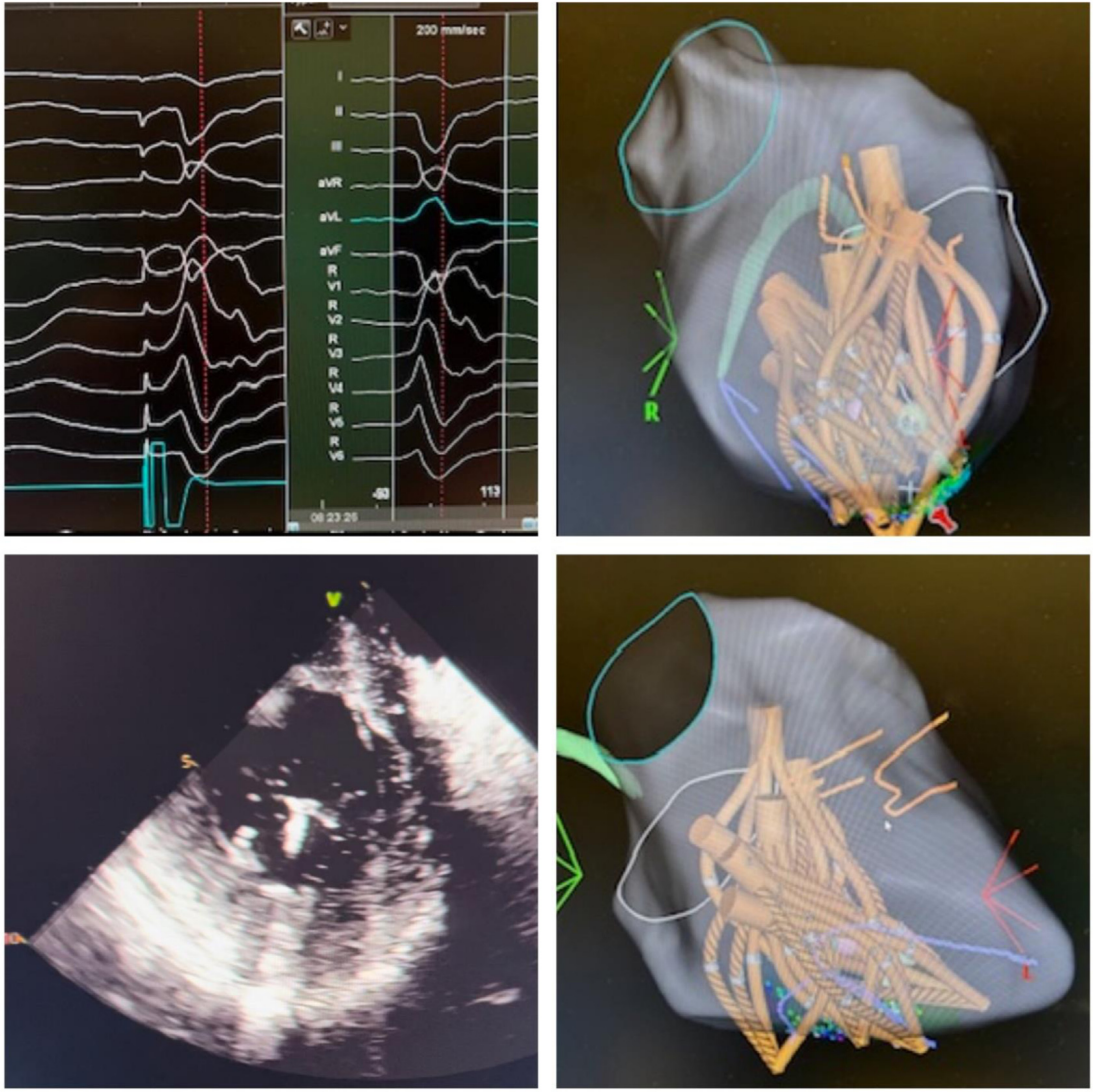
Pace mapping through the FARWAVE catheter. The Farawave catheter in basket configuration on the papillary muscle in left anterior oblique and right anterior oblique views. Intracardiac echocardiography image of the catheter on the papillary muscle.
